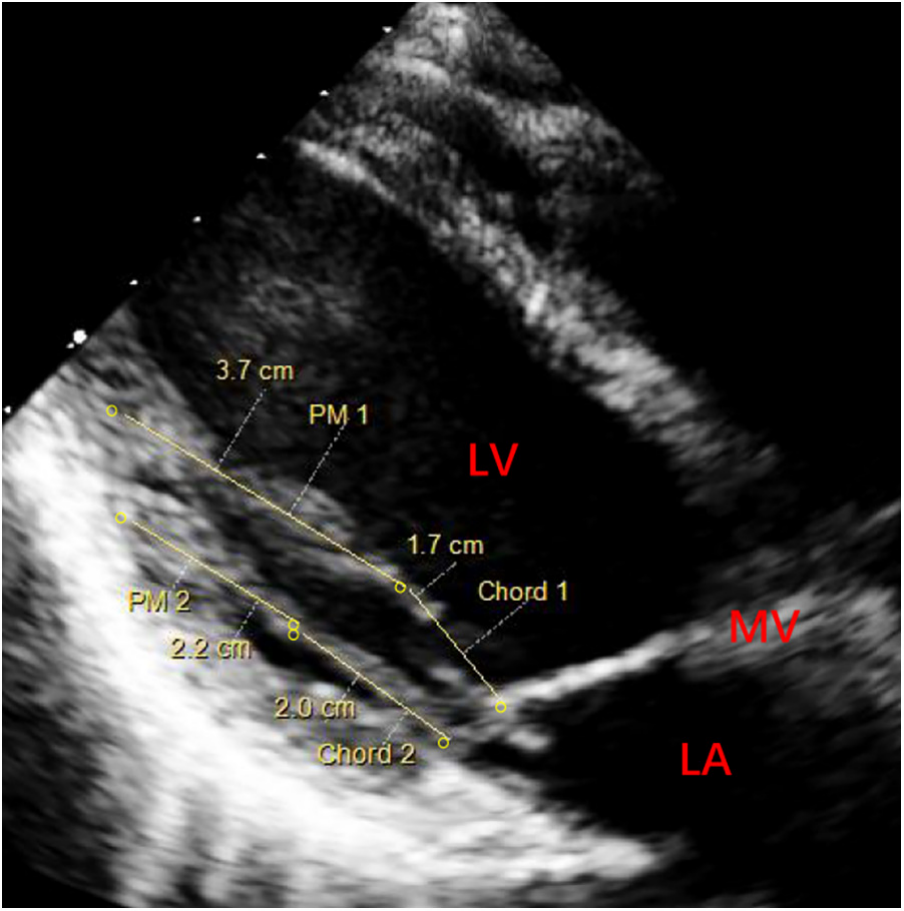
Preoperative echocardiography (parasternal long-axis view) of left ventricular systole for measuring the length of papillary muscles. PM 1 (elongated PM, 3.7 cm) and Chord 1 (the chord of abnormal PM, 1.7 cm). PM 2 (normal PM, 2.2 cm) and Chord 2 (normal chord, 2.0 cm). PM, papillary muscle; MV, mitral valve; LA, left atrium; LV, left ventricle.
